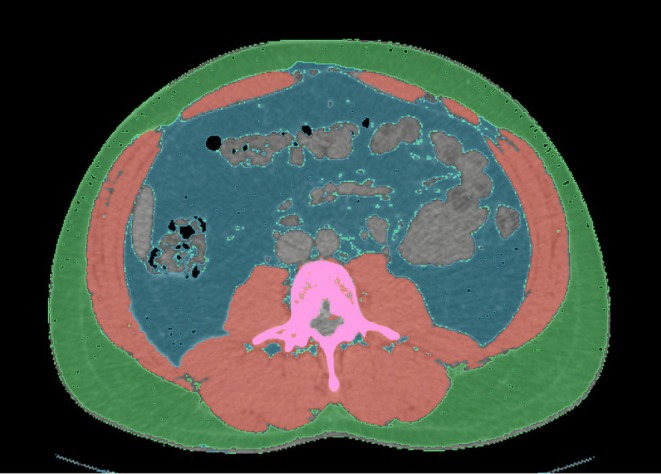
Segmentation of a CT axial image at the L3 lumbar vertebra level using Hounsfield units (HU): Subcutaneous adipose tissue is identified with a threshold between −190 and −30 HU (green), visceral adipose tissue between −150 and −50 HU (blue) and skeletal muscle tissue between −29 and 150 HU (red). Higher HU values (> 400) can detect bone (pink). The segmentation is performed with an open‐source software (http://www.slicer.org, version 5.6.2, revision 32448, built 2024‐04‐05).
